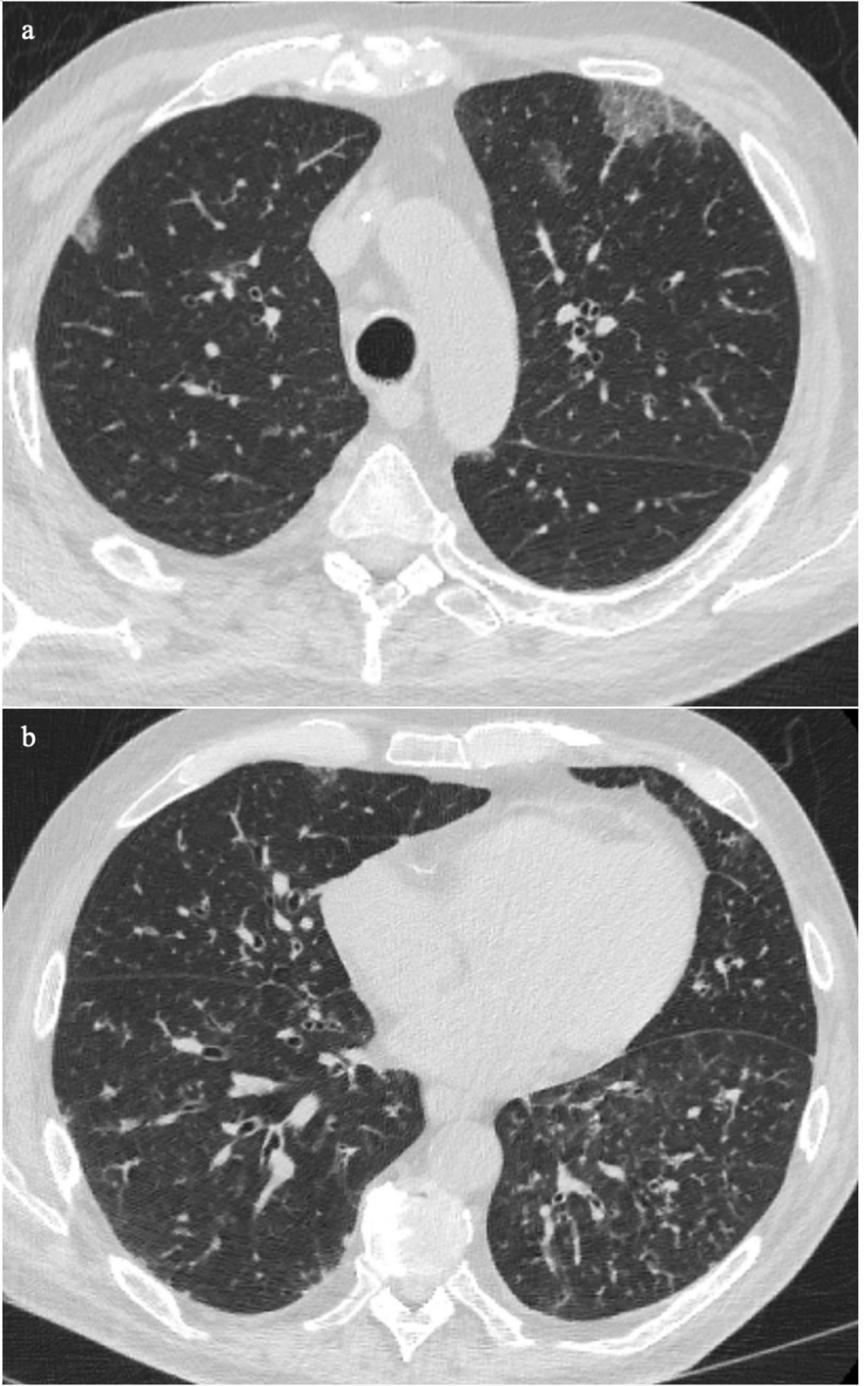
This patient is immunosuppressed with cough and fever. The CT showed peripheral crazy paving in the upper lobes, which is supportive of COVID-19 infection (8a). The bronchiectasis and mucus plugging in the lower lobes are typical of bacterial infection (8b)
